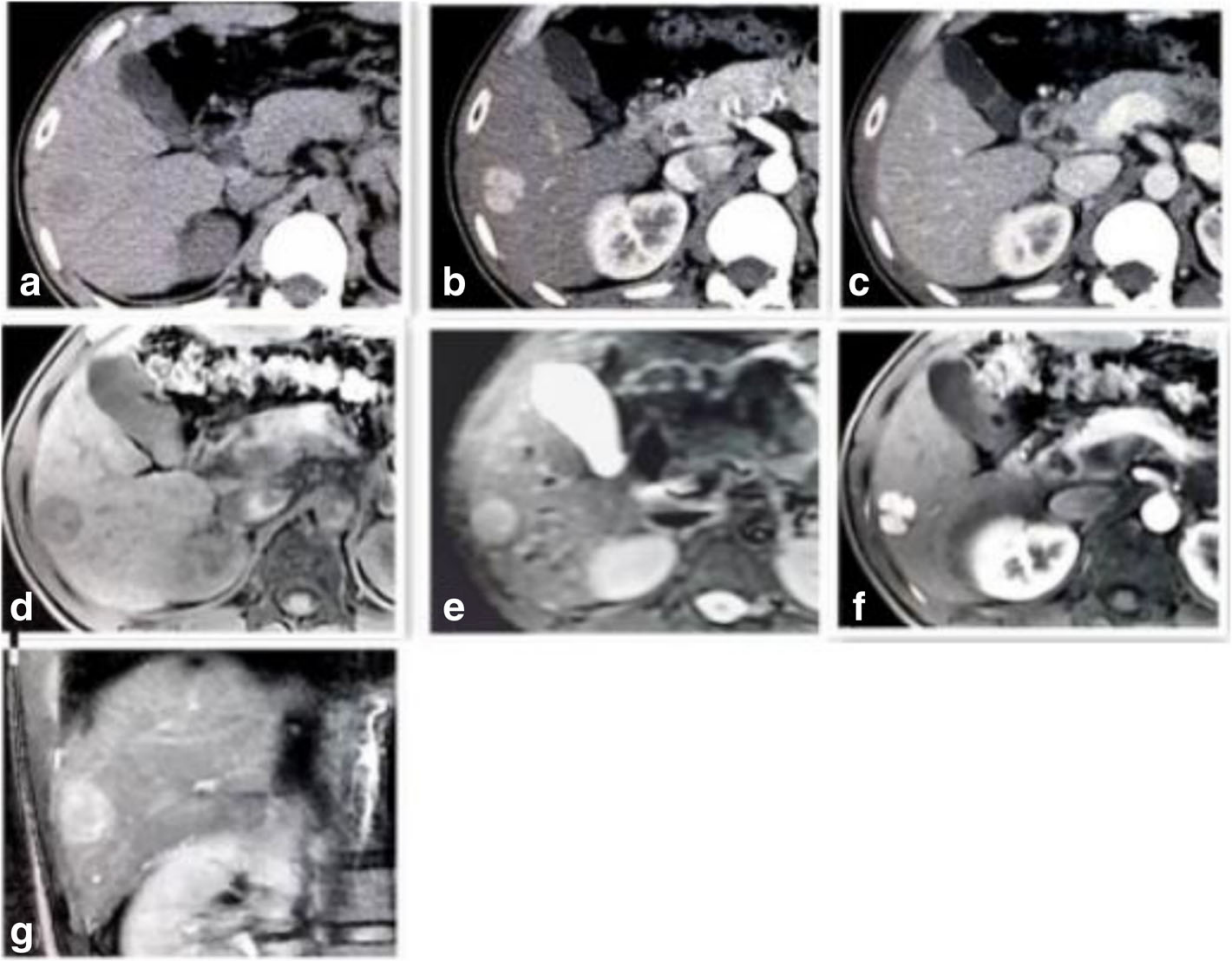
CT scan axial images (a-c) showing a hypodense liver mass in the right lobe, which demonstrated significant enhancement in the arterial phase and peripheral rim enhancement in the delayed phase. MR images (d-g), T1-weighted image (d): lesion shows well circumscribed, heterogeneous, and hypointense masses. T2-weighted axial image (e) shows well-circumscribed, high signal intensity masses. On enhanced MR images, lesions show significant enhancement and tumor lobulation in the early arterial phase (f), enhancement in the 5 min delayed image (g)
